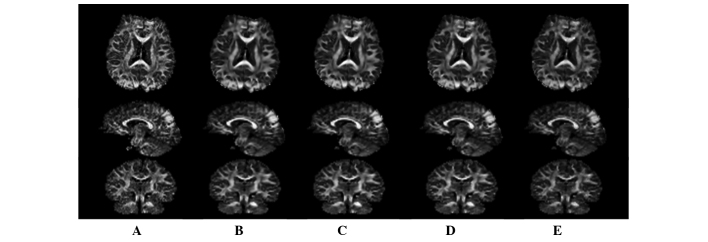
In vivo* FA maps prior to and following denoising. FA map of (A) original image, and denoising with (B) RD, (C) LED and (D) ED weighting and (E) UNLM in DWI space. Upper row, axial plane; middle row, sagittal plane; lower row, coronal plane; FA, fractional anisotrophy; RD, Riemannian distance; LED, Log-Euclidean distance; ED, Euclidean distance; UNLM, unbiased non-local mean; DWI, diffusion-weighted image.
